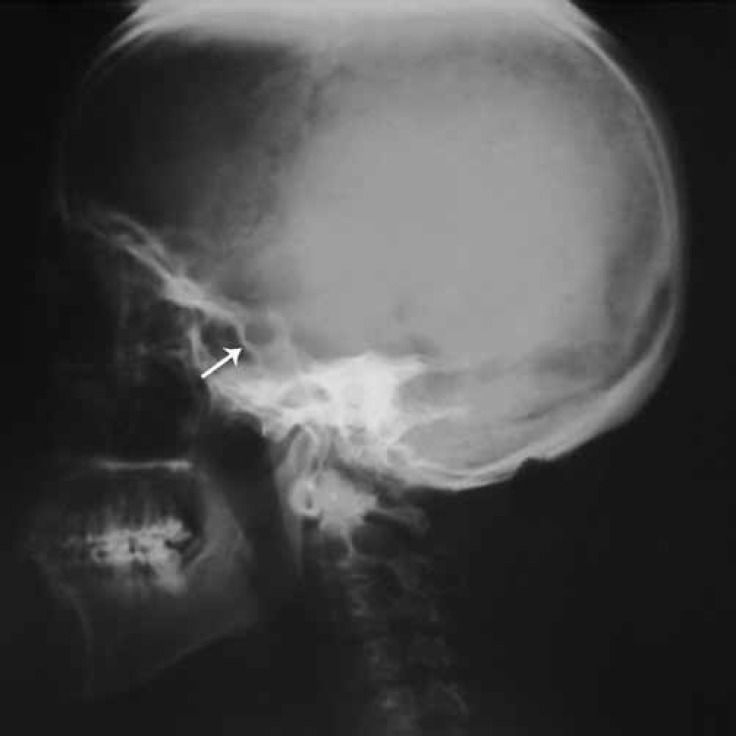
Lateral view X-ray of the patient’s skull shows J-shaped sella turcica (white arrow).
